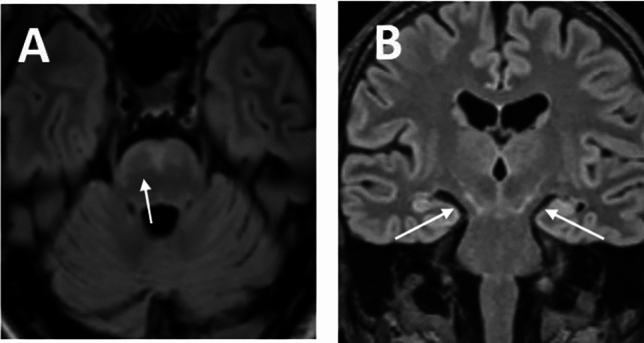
Cerebral MRI in CNS-LNB. (A) ‘Rounded M’-sign with T2-hyperintense pontine lesion in a patient with encephalomyelitis. (B) subtle ‘Tarsier-sign’ with T2-hypertintense mesencephalic lesion at the cerebral peduncles in the same patient. White arrows depict representative findings.
